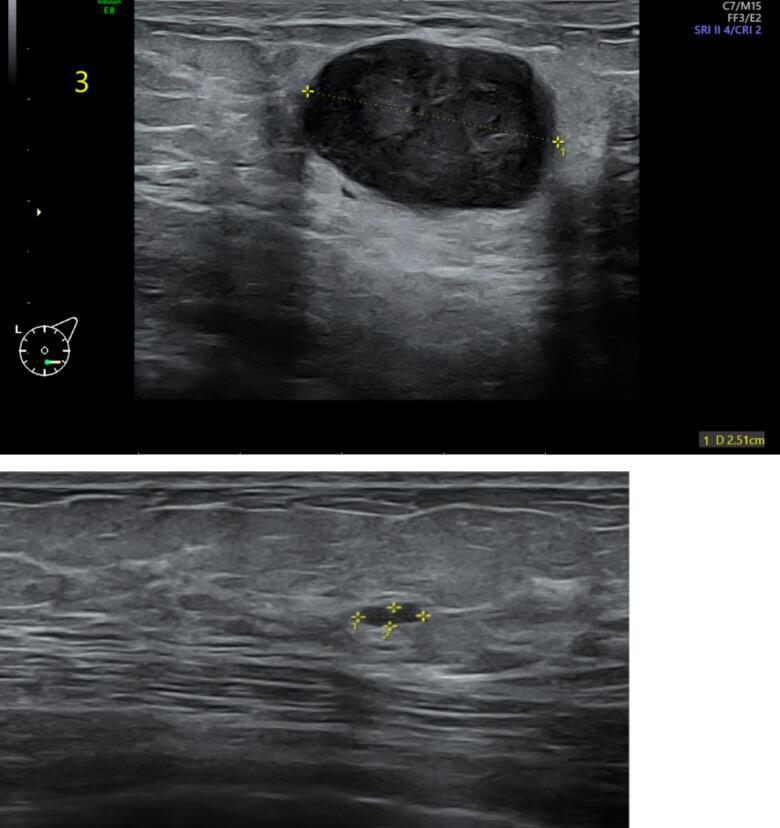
Ultrasound of the left breast showing breast mass at the 6:00 O'clock position, located 3 cm away from the nipple, 2.6 × 2.1 × 2.4 cm in size with heterogenous echotexture and posterior acoustic enhancement meriting a BI-RADS 4 classification.
